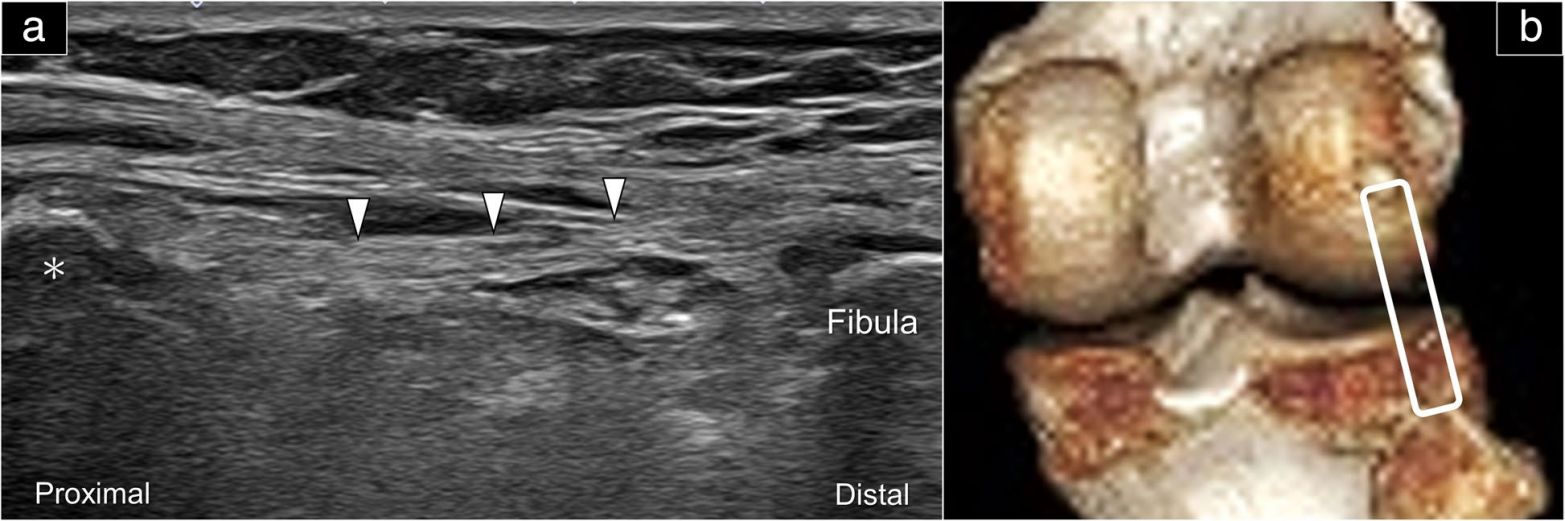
Sonographic imaging of the fabellofibular ligament in the long-axis view (a). The colored dashed rectangles indicate the transducer’s positions (b). White arrowhead: fabellofibular ligament; asterisk: fabella
